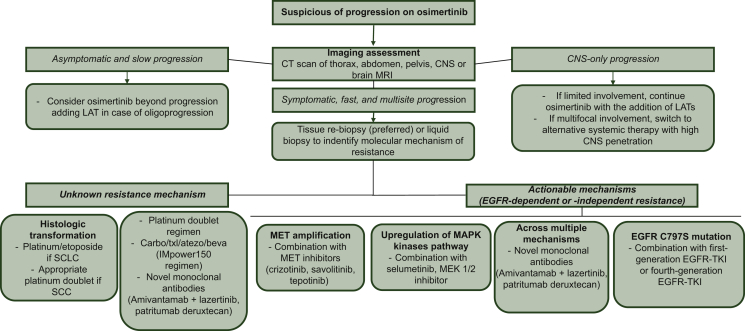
Proposed algorithm for managing epidermal growth factor receptor (EGFR)-mutated non-small-cell lung cancer progressing on osimertinib. CNS, central nervous system; CT, computed tomography; LAT, locally ablative therapy; MRI, magnetic resonance imaging; SCC, squamous cell carcinoma; SCLC, small-cell lung cancer; TKI, tyrosine kinase inhibitor.
